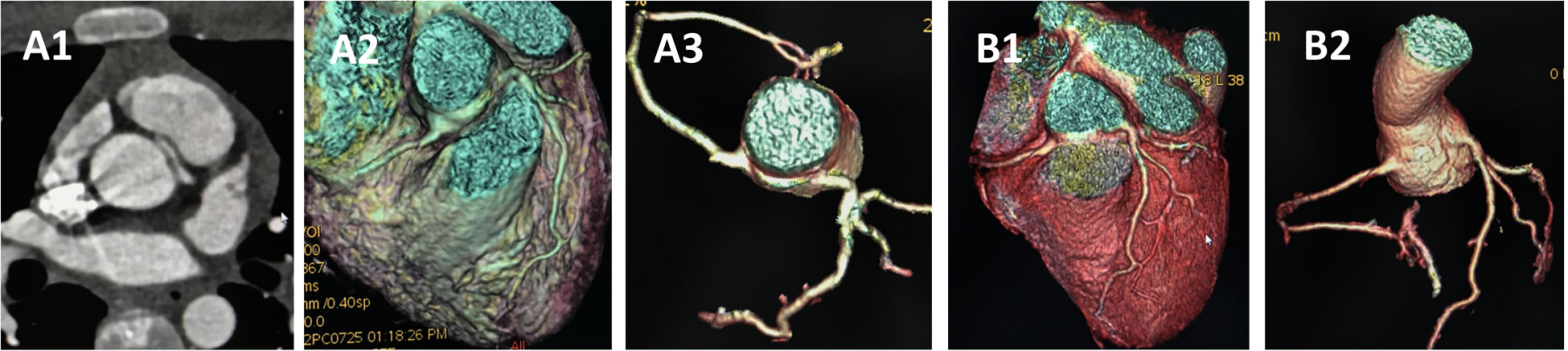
CCTA of case 2 underwent unroofing. (A) Preoperative images showing anomalous left coronary artery arising from the right sinus. (B) Follow-up images showing proximal left main coronary artery without visible stenosis. CCTA, coronary computed tomography angiography.
